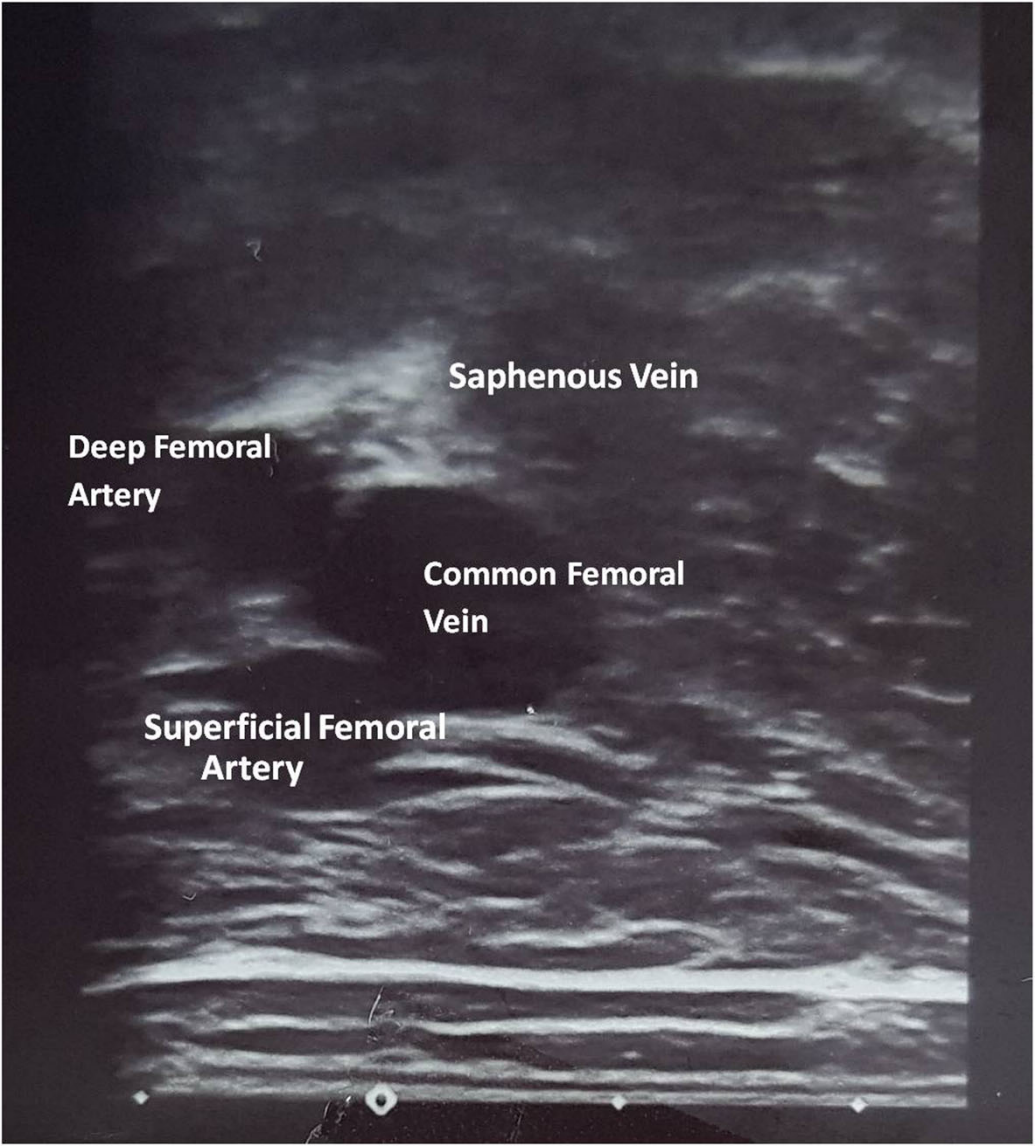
Doppler ultrasound images of the lower right limb showing a superficial vein thrombosis (SVT). Anterior accessory saphenous vein (AASV), great saphenous vein (GSV), common femoral vein (CFV), and common femoral artery (CFA) in the Doppler ultrasound images of the lower right limb
